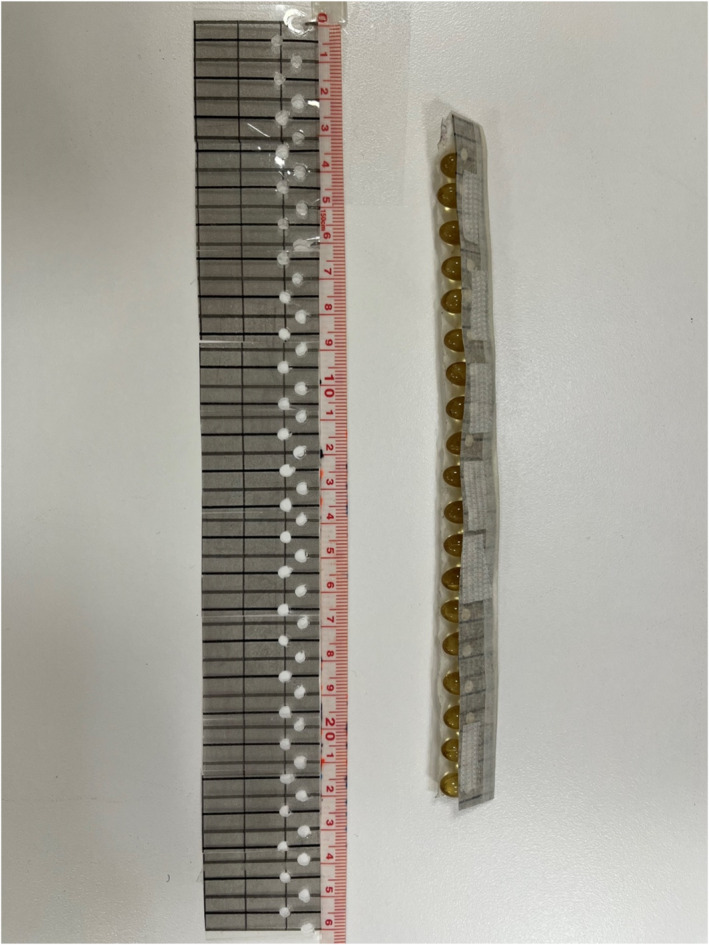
Gel capsule strip used for MR imaging and marker template for marking feet for imaging.
